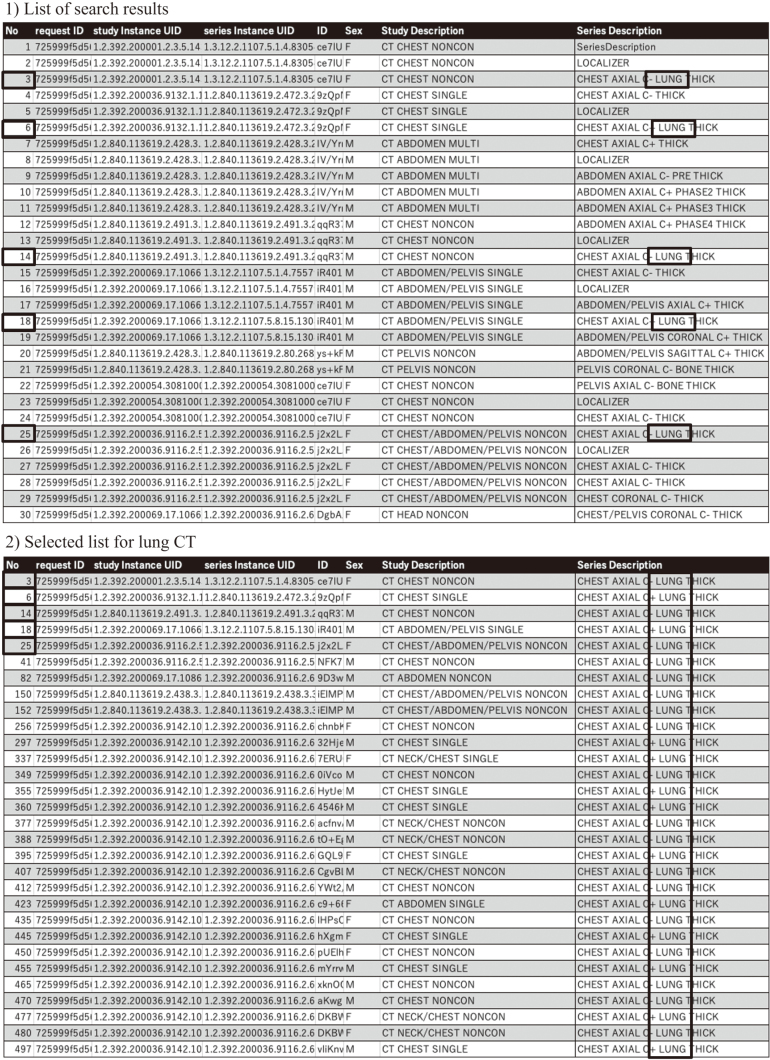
New file-download system 1) Creating search results in CSV file format is possible on the J-MID cloud. 2) After data cleansing the list to select the series of CT images with a lung window (for example), the selected series within the list can be obtained by uploading the list to the J-MID system.
